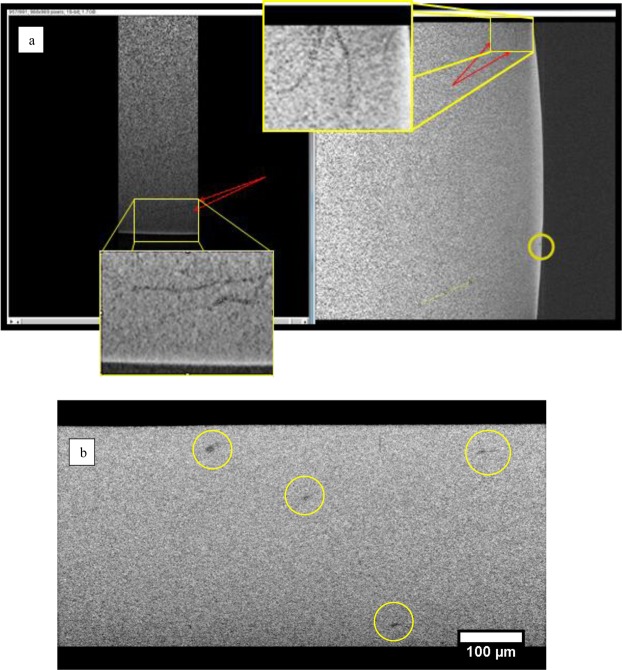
µ-CT showing (a). Base view (left) and Right view (right) exhibiting a multiple pathed crack propagating parallel to the surface of the H_2_ ball, (b). Pores in the H_2_ race wear track subsurface.
